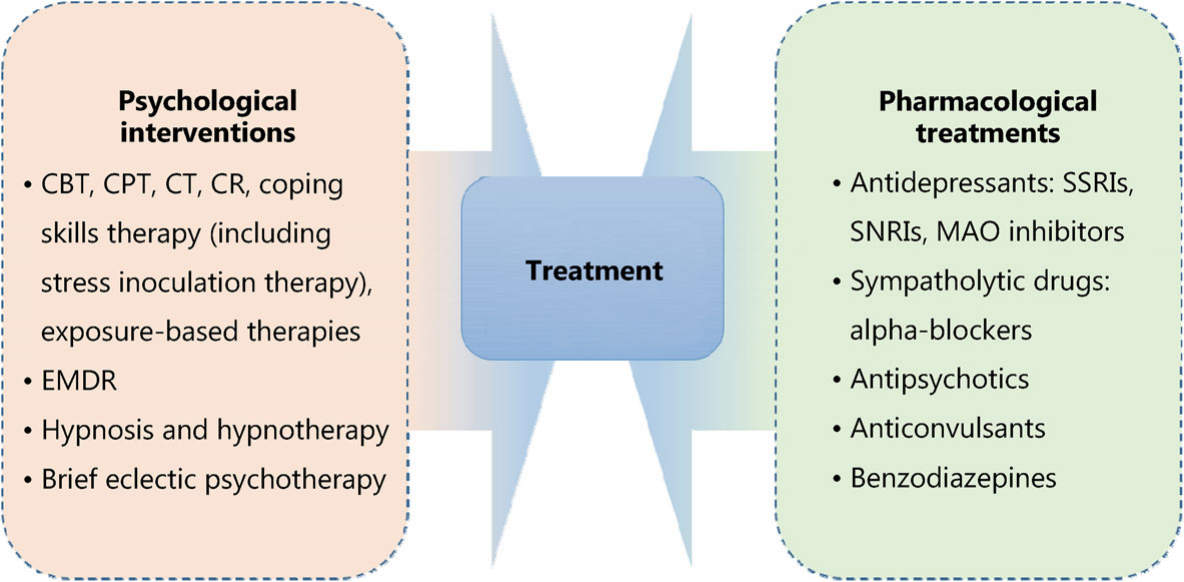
Psychological and pharmacological strategies for treatment of PTSD. CBT. Cognitive behavioral therapy; CPT. Cognitive processing therapy; CT. Cognitive therapy; CR. Cognitive restructuring; EMDR. Eye movement desensitization and reprocessing; SSRIs. Selective serotonin reuptake inhibitors; SNRIs. Serotonin and norepinephrine reuptake inhibitors; MAO. Monoamine oxidase
